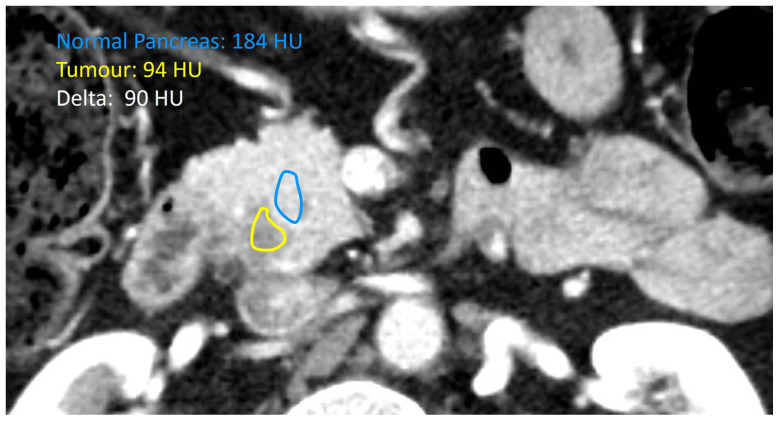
Representative CT slice for one of our biophysical subtype analyses of pancreatic ductal adenocarcinoma showing the interface between tumour and normal pancreatic parenchyma, with the resulting deltaHU (Hounsfield Unit).
